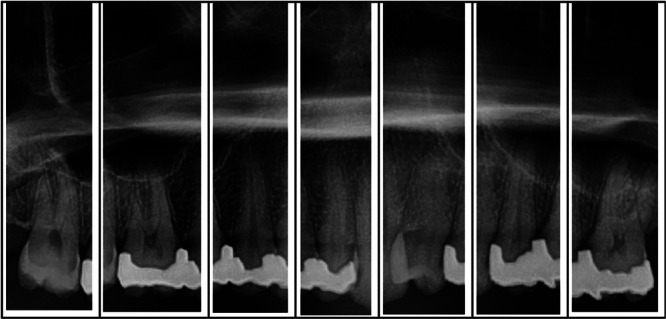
Sectional view of the posterior maxillary teeth in panoramic radiography.
